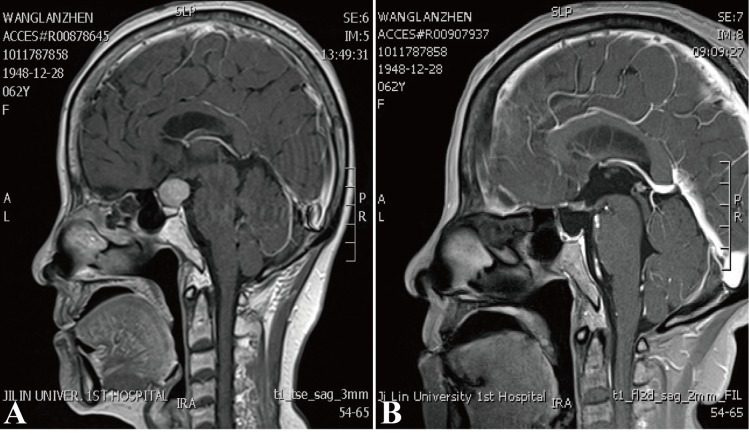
MRI images of case 2. (A) Preoperative MRI image demonstrating a suprasellar mass. (B) MRI image at the 15-month follow-up revealing no recurrence of the tumor. MRI, magnetic resonance imaging.
